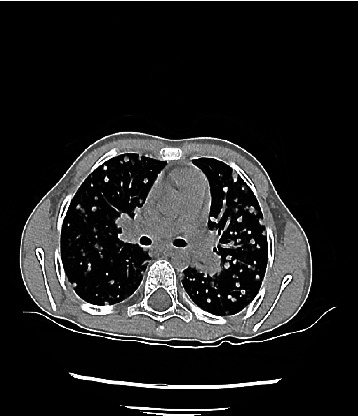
Multiple pulmonary nodules in both lungs on CT.
